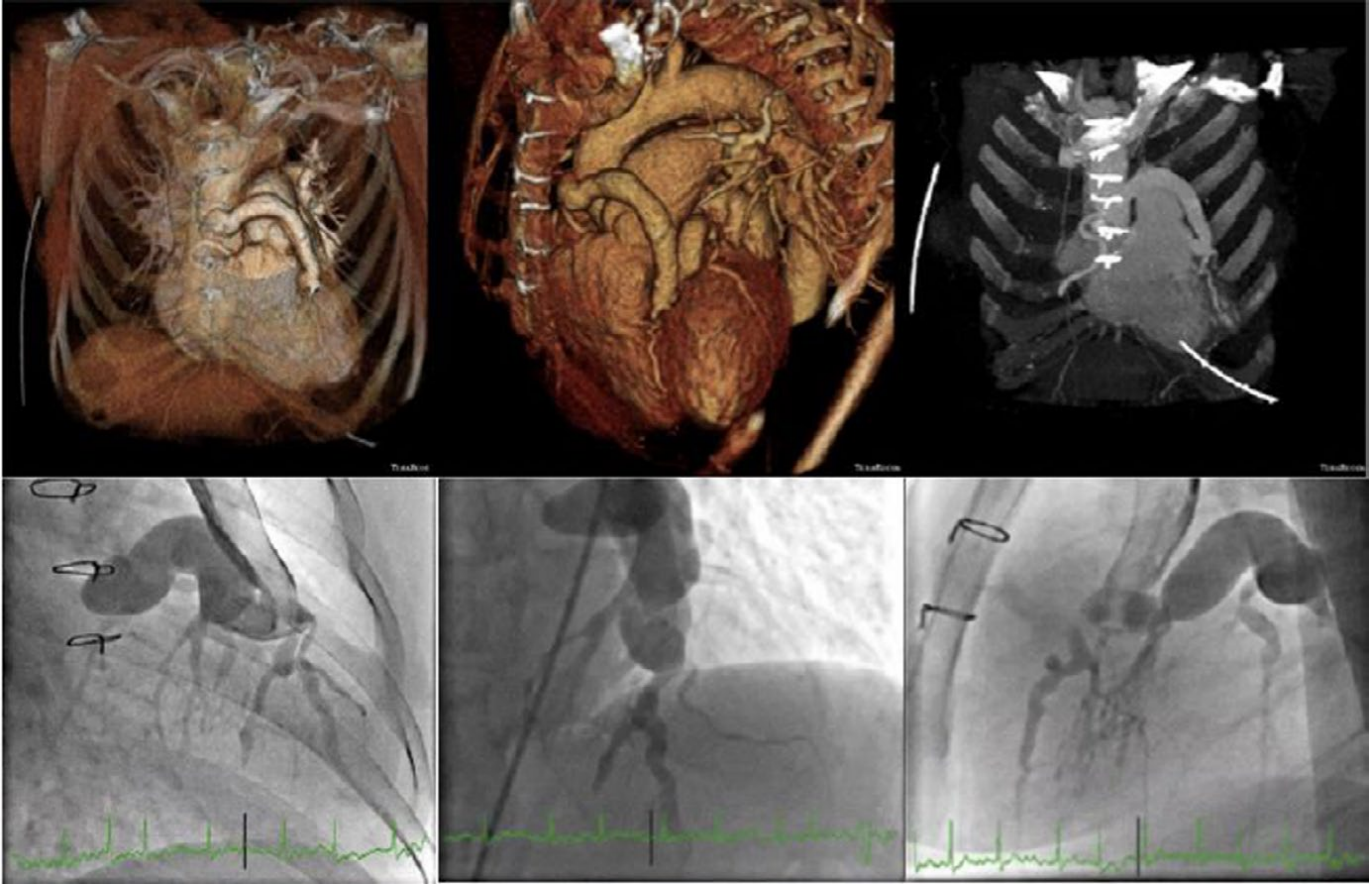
Computed tomography and coronary angiography showing the dilated patent coronary graft (saphenous vein to left anterior descending artery)
